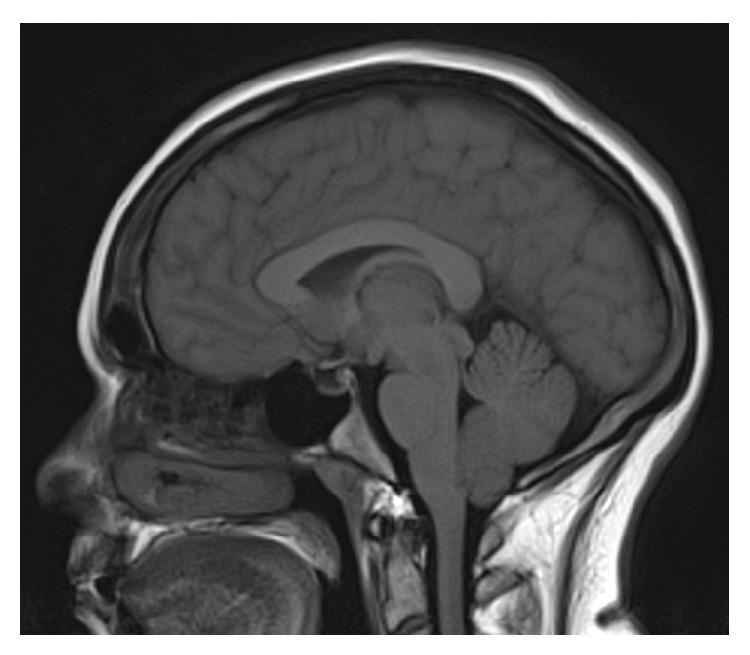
Sagittal MRI at diagnosis demonstrated 4 mm of cerebellar tonsillar descent.
